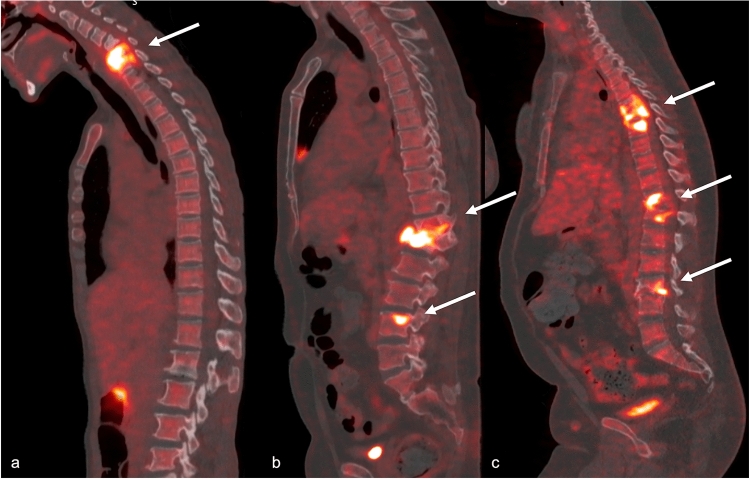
PET/CT-imaging of three patients with different amounts of spinal lesions*. a Patient with three non-contiguous spinal lesions, b Patient with two non-contiguous spinal lesions, c Patient with singular spinal lesion
